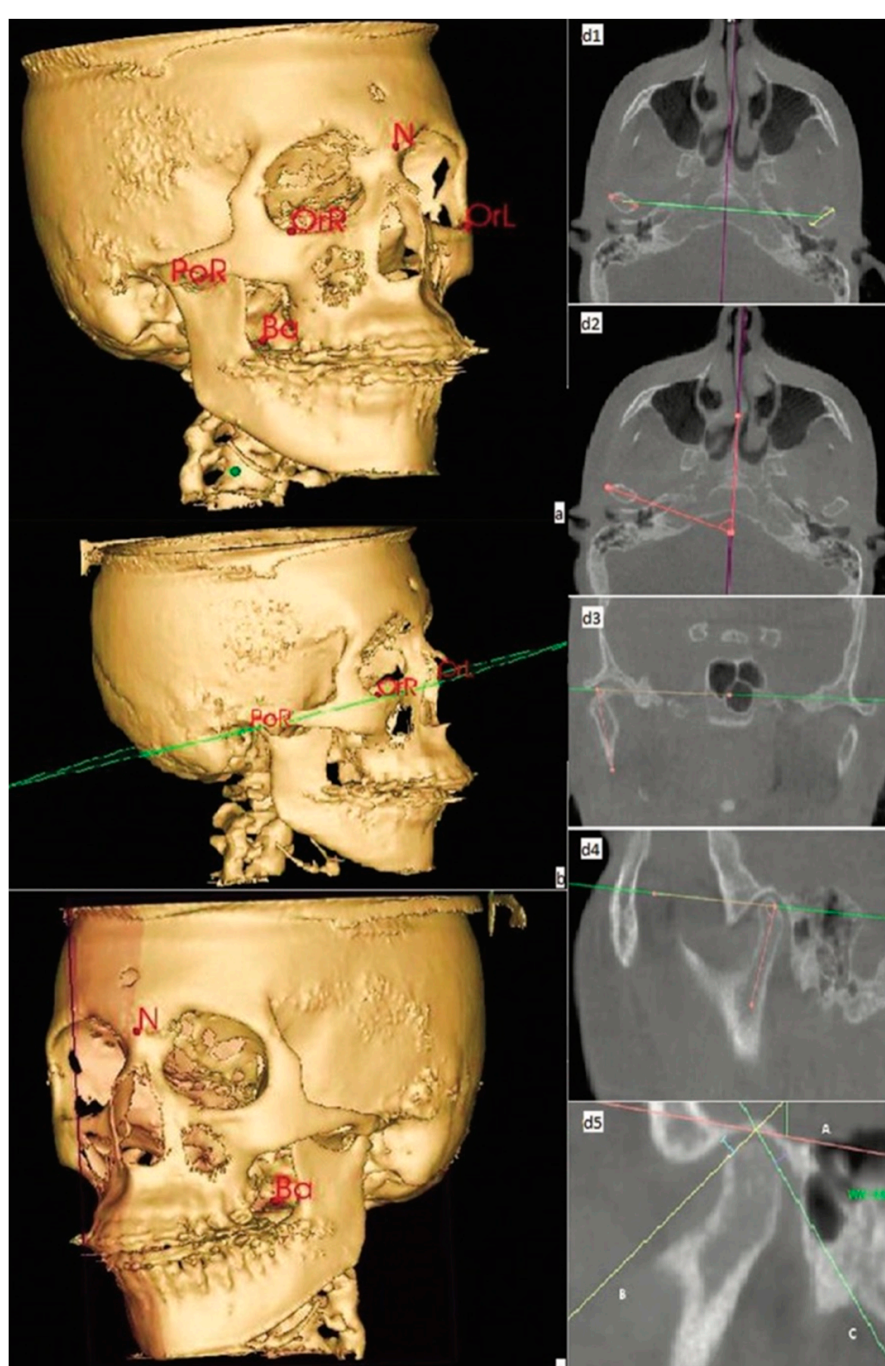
CBCT image evaluation: (a) midpoints of reference; (b) Frankfort horizontal plane; (c) midsagittal plane; (d1) intercondylar distance; (d2) condylar angulation in the axial plane; (d3) condylar angulation in the frontal plane; (d4) condylar angulation in the sagittal plane; (d5) condylar position in the sagittal plane. Adapted from Vale et al. [23].
